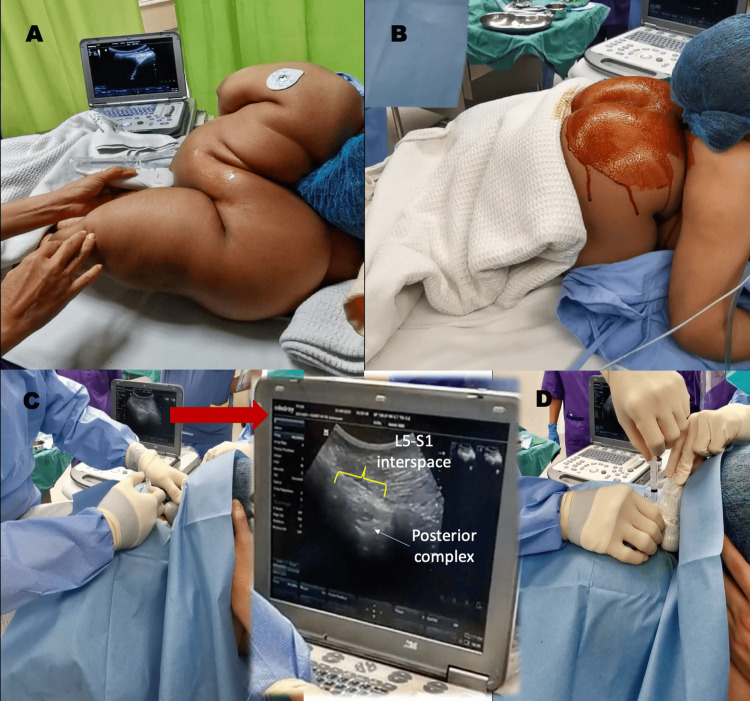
Technical challenges in performing neuraxial anesthesia A. The patient could not sit up properly, and the room for scanning plus needling in a sitting position was extremely limited. B. The patient turned to a prone kneeling position for a neuraxial technique. C. Attempting continuous spinal anesthesia under real-time ultrasound guidance using the Sprotte needle 23G (Pajunk Intralong, Geisingen, Germany). The needle was inserted in-plane caudal-to-cranial needling technique; Inset: Ultrasound of the lumbar spine (paramedian oblique sagittal view) showed L5-S1 intervertebral space and posterior complex. D. However, there was negative efflux of cerebrospinal fluid (CSF) through the spinal hub despite waiting for a minute and negative aspiration. A spinal test dose of levobupivacaine 0.5% 0.5 mL was delivered, but the patient did not experience progressive numbness or weakness of the bilateral lower limb, thus the neuraxial technique was abandoned.
